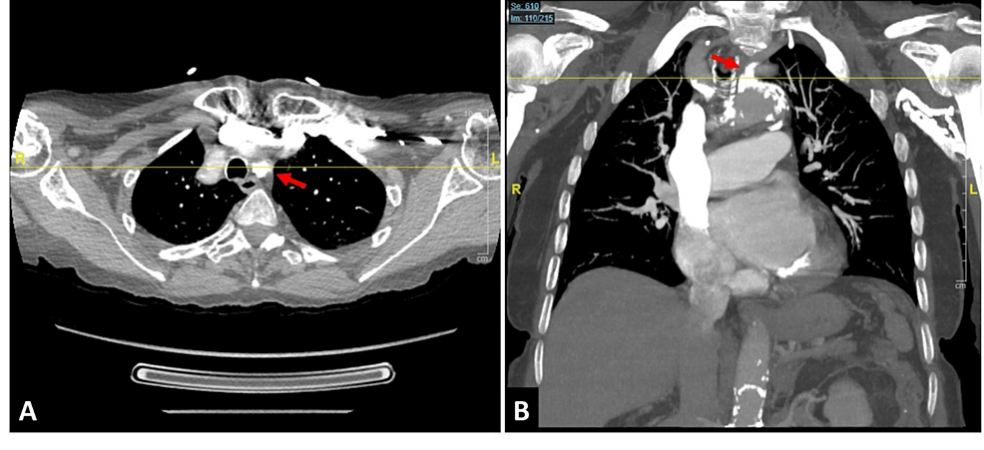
CTA chest. High-grade short-segment stenosis of origin of left subclavian artery (red arrow), near the take-off point from the aorta in axial (A) and coronal (B) views. Of note, post-stenotic segments of the left subclavian are patent. CTA, computed tomography angiography.
